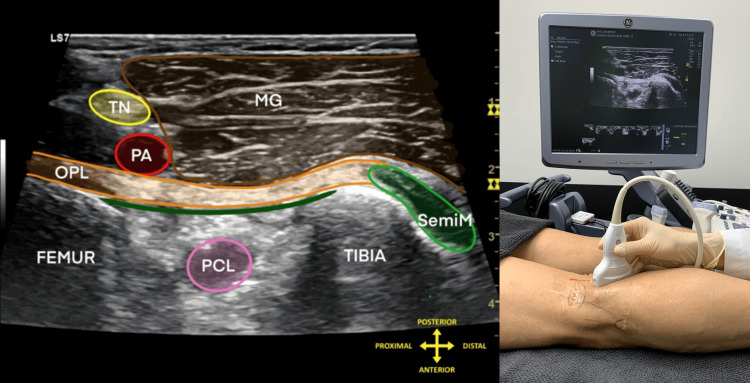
Oblique transverse ultrasound scan demonstrating the oblique popliteal ligament Oblique transverse ultrasound image obtained over the posteromedial knee demonstrating the oblique popliteal ligament within the posterior capsuloligamentous complex. This view serves as an intermediate orientation step between the initial transverse safety scan and the more target-specific longitudinal view. The corresponding probe position and hand position are shown to facilitate reproducibility.
